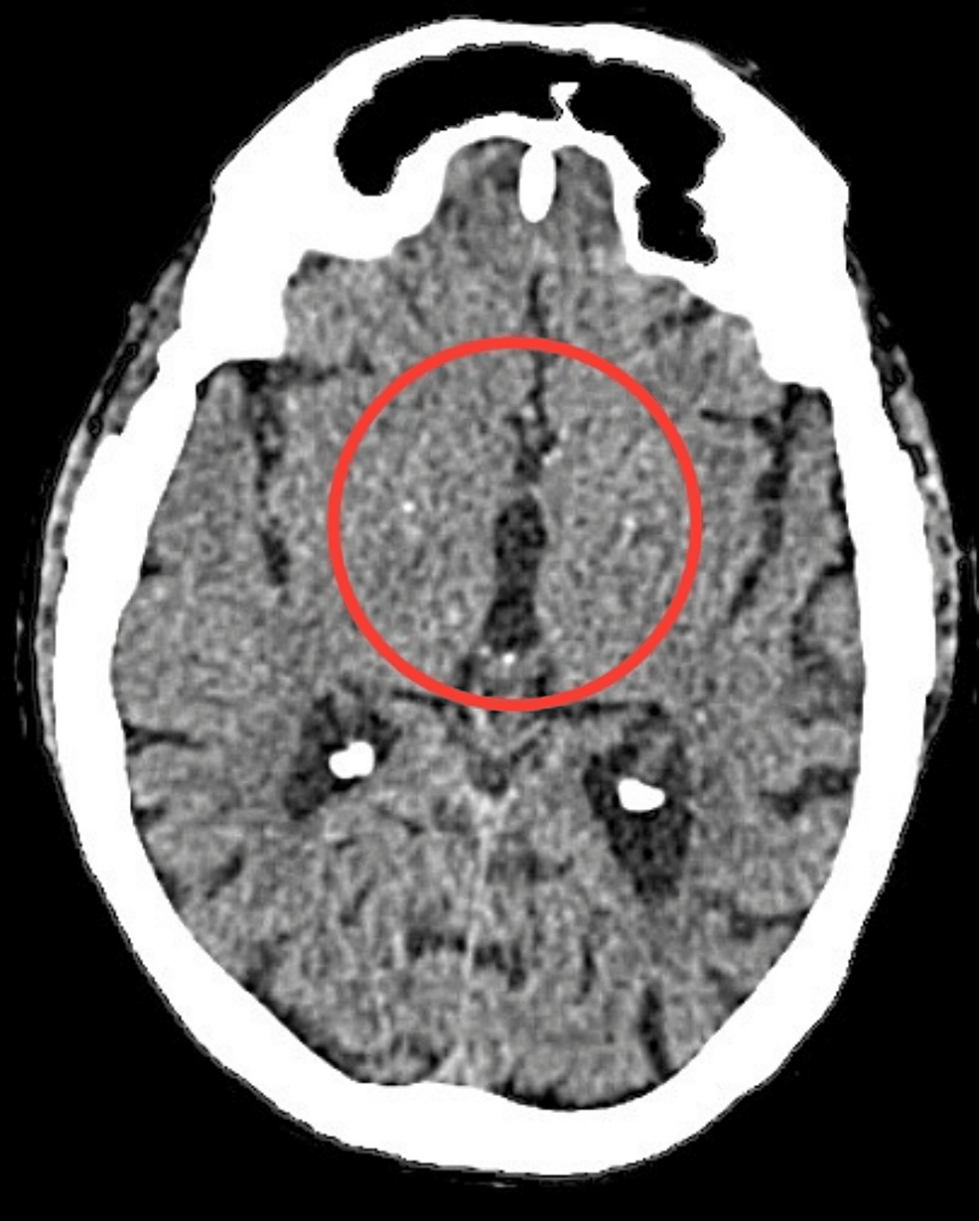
CT scan of the brain The red circle indicates chronic lacunar infarcts in the left gangliocapsular regions.
